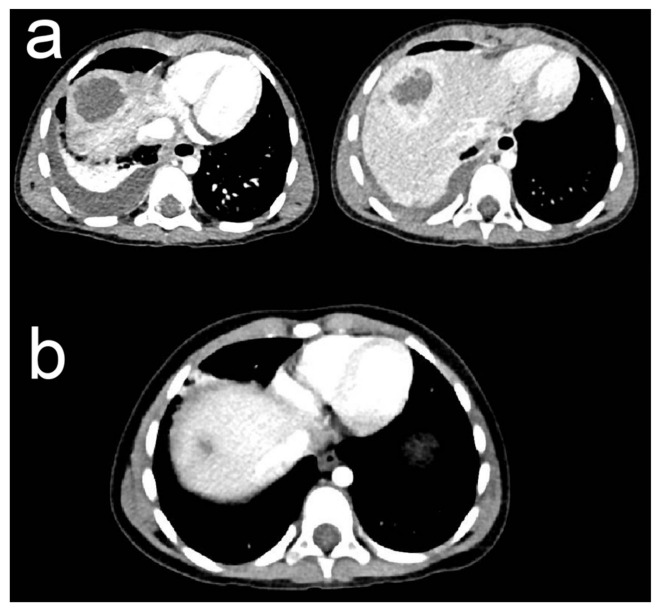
Abdominal Computed Tomography (CT). (a) Before treatment—the abdominal CT image showing 2 hypodense collections (size: 39 × 35 × 36 mm and 22 × 20 × 23 mm) on segment VIII of the liver, suggestive of liver abscesses. (b) After treatment—the abdominal CT scan reveals the evolution of the hepatic abscesses to a fibrous scar, suggesting the remission of the lesion.
